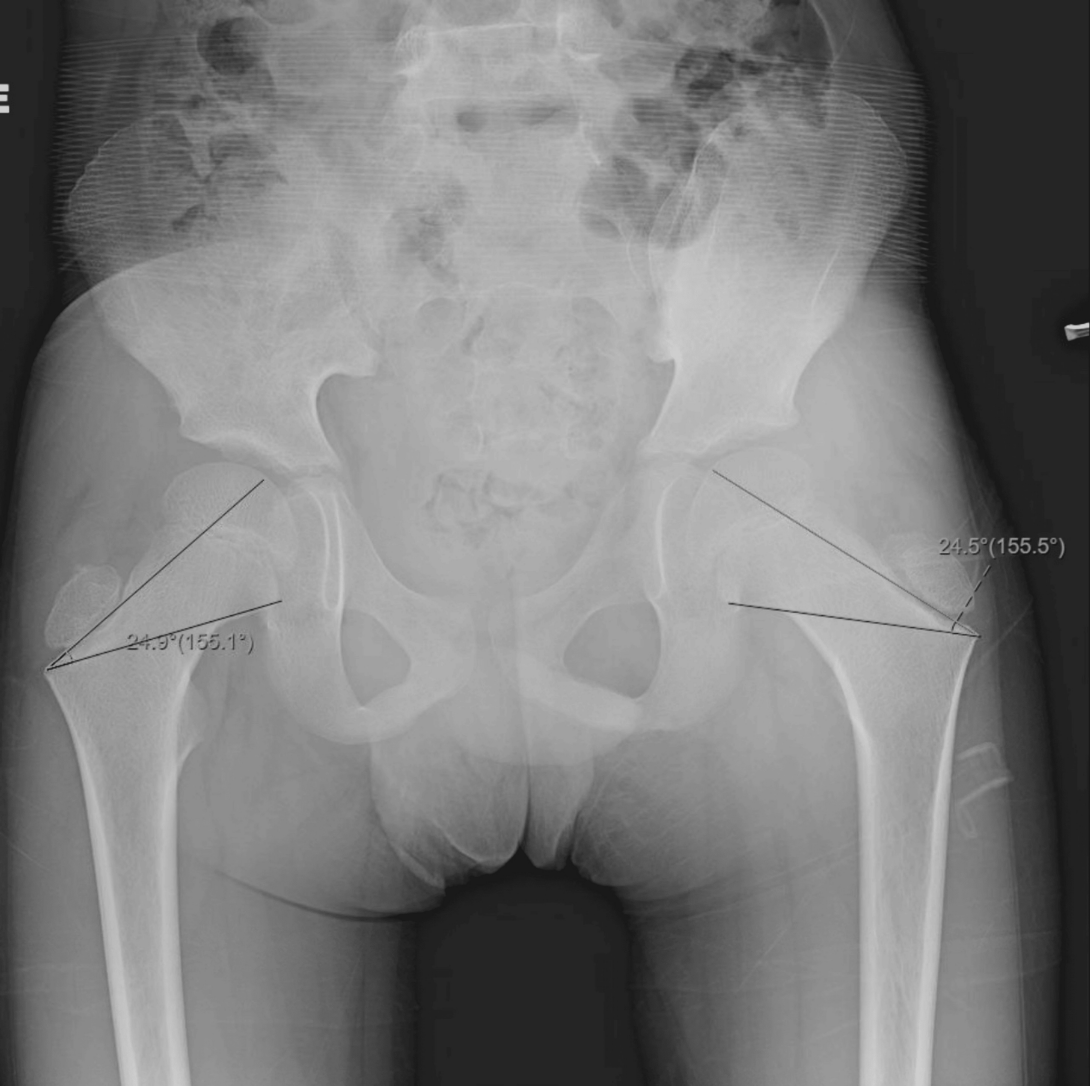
Hip X-ray showing asymmetric prominence of the left greater trochanter physis
